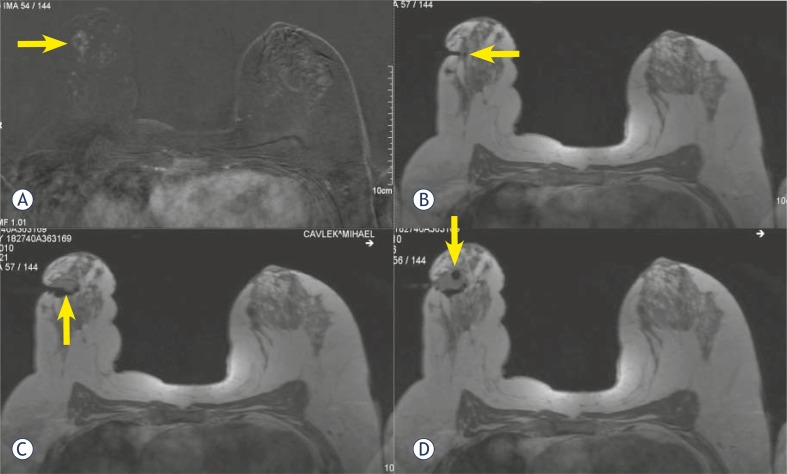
MRI-guided biopsy using axial, contrast-enhanced FLASH 3D T1W images. The pre-biopsy fat-suppressed image (A) shows an enhancing lesion in the right breast. The biopsy needle (B) is seen after localization, along with the lesion. Following the biopsy (D) the small magnetic susceptibility artefact due to the clip in situ is seen.
